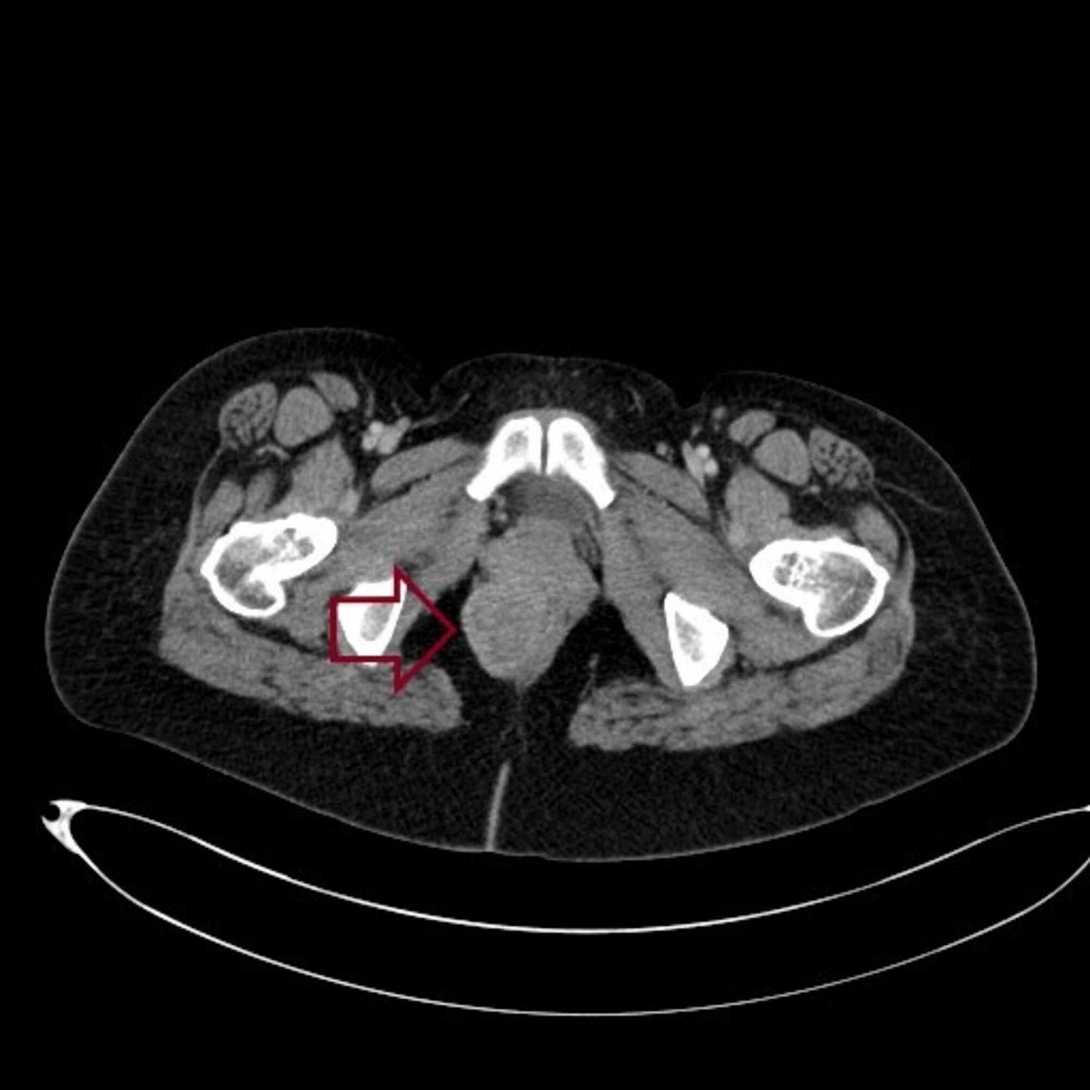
Pelvic computed tomography showing a mass of the right lateral rectal wall (red arrow)
